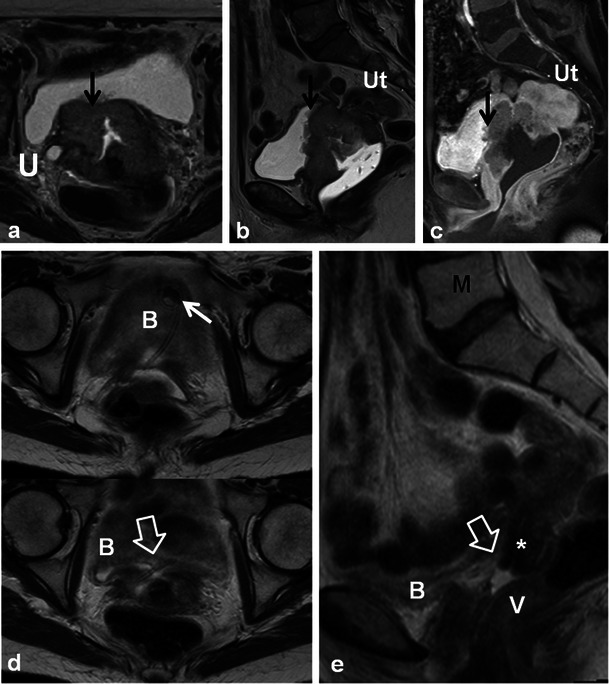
A 57-year-old woman with locally advanced (Stage IVa) squamous cell carcinoma of the cervix demonstrating invasion of the posterior wall of the urinary bladder (*black arrow*) and right ureter (*U*) at baseline MRI on axial and sagittal T2 TSE (a and b) and sagittal T1 fat-saturated post-gadolinium (c) gradient recalled echo (GRE) images. Note sterile ultrasound gel within vagina and atrophic uterus (*Ut*). Following RT there is a small but definite communication between the anterior wall of the vagina and the posterior wall of the urinary bladder (*open arrows*) depicted on axial (d) and sagittal (e) T2 TSE findings consistent with post-RT vesicovaginal fistula. A double-J ureteric stent (*short white arrow*) to relieve right hydroureteronephrosis and fatty marrow replacement (*M*) are also noted
